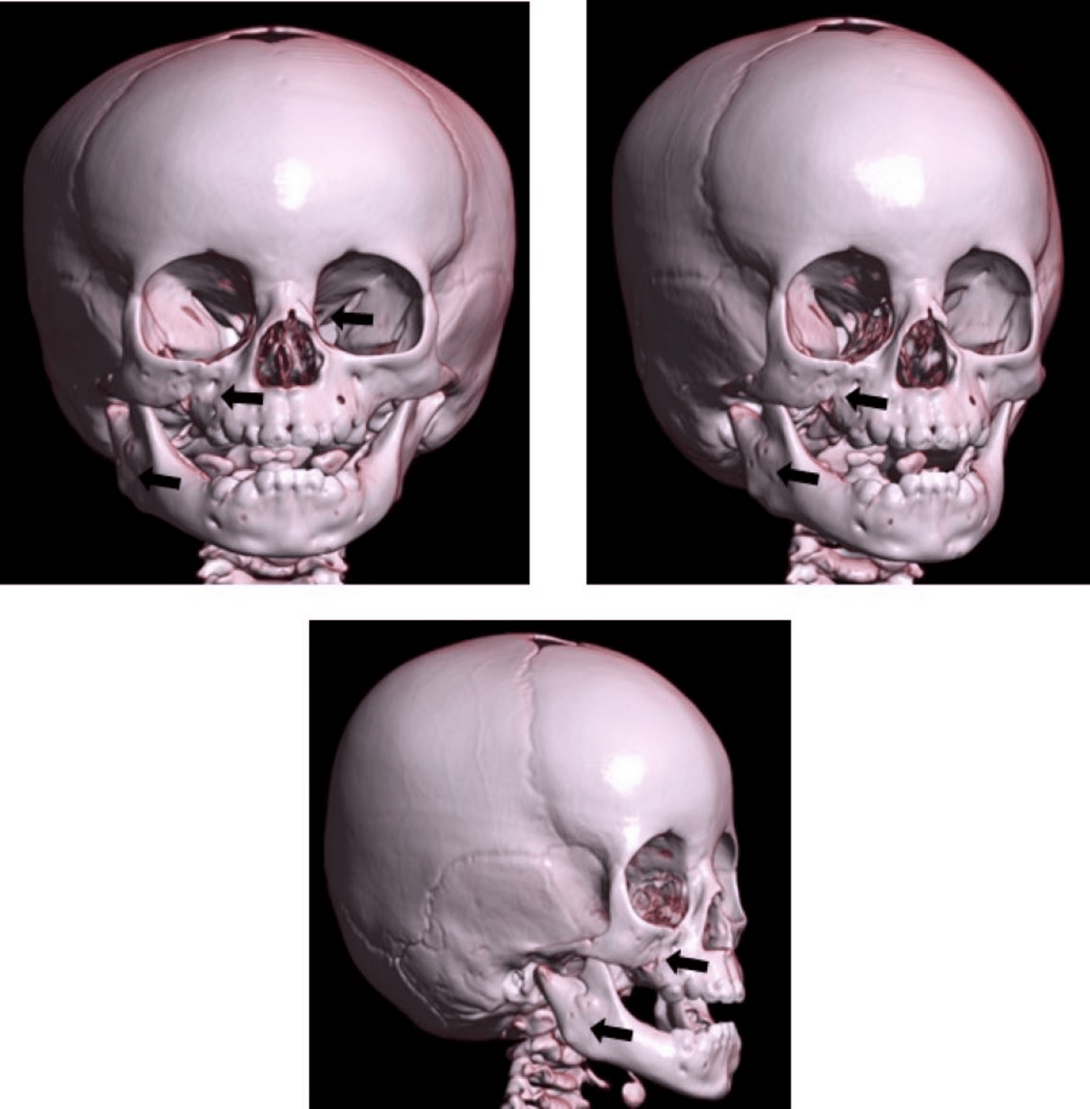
Tomographic reconstruction: postsurgical images Simple 3D CT reconstruction; reduced condylar Kohler V, orbitomalar right Knight North V, right orbital fracture, unicortical symphyseal, and nasal fractures with absorbable polylactic acid plates CT: computed tomography
